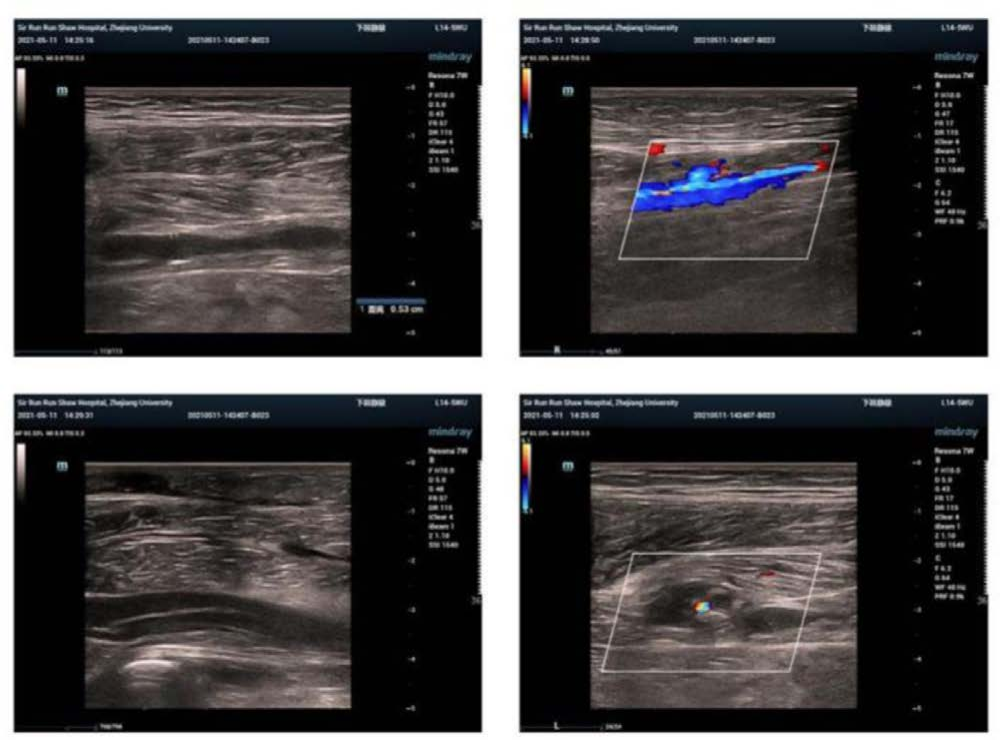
Venous duplex ultrasonography confirmed multiple thrombosis of the left lower extremity intermuscular vein.
